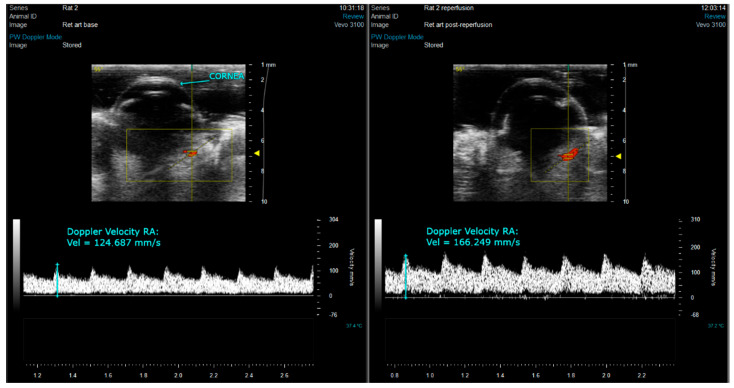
Representative ultrasound images showing blood supply of the eye bulb before and after ischemic ligation. Yellow box: Area of color doppler imaging. Vertical yellow line with short horizontal parallel yellow lines in the middle: gate of pulsed wave doppler (between the two short horizontal lines). Yellow dashed transverse line: angle of pulsed wave doppler. Red: Bloodflow towards the transducer. Blue line: measuring tool for maximal bloodflow velocity.
